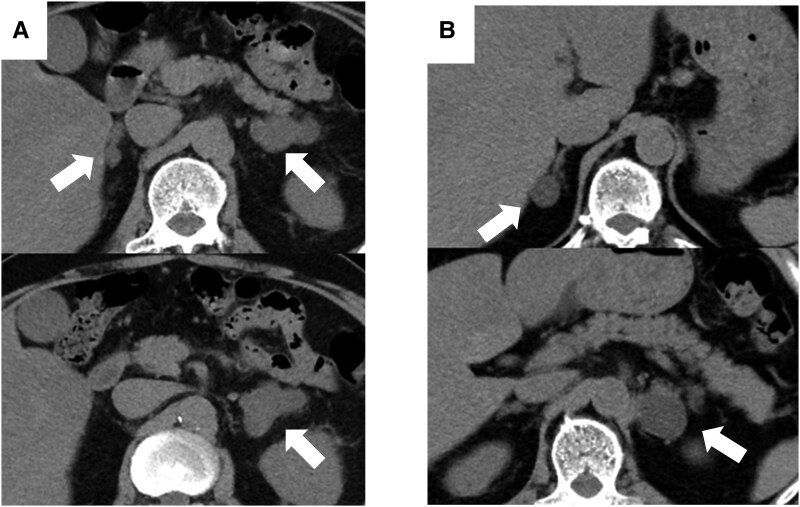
Adrenal nodules. (A) Abdominal computed tomography (CT) scan of case 1. (B) Abdominal CT scan of case 2. Arrows indicate adrenal nodules.
